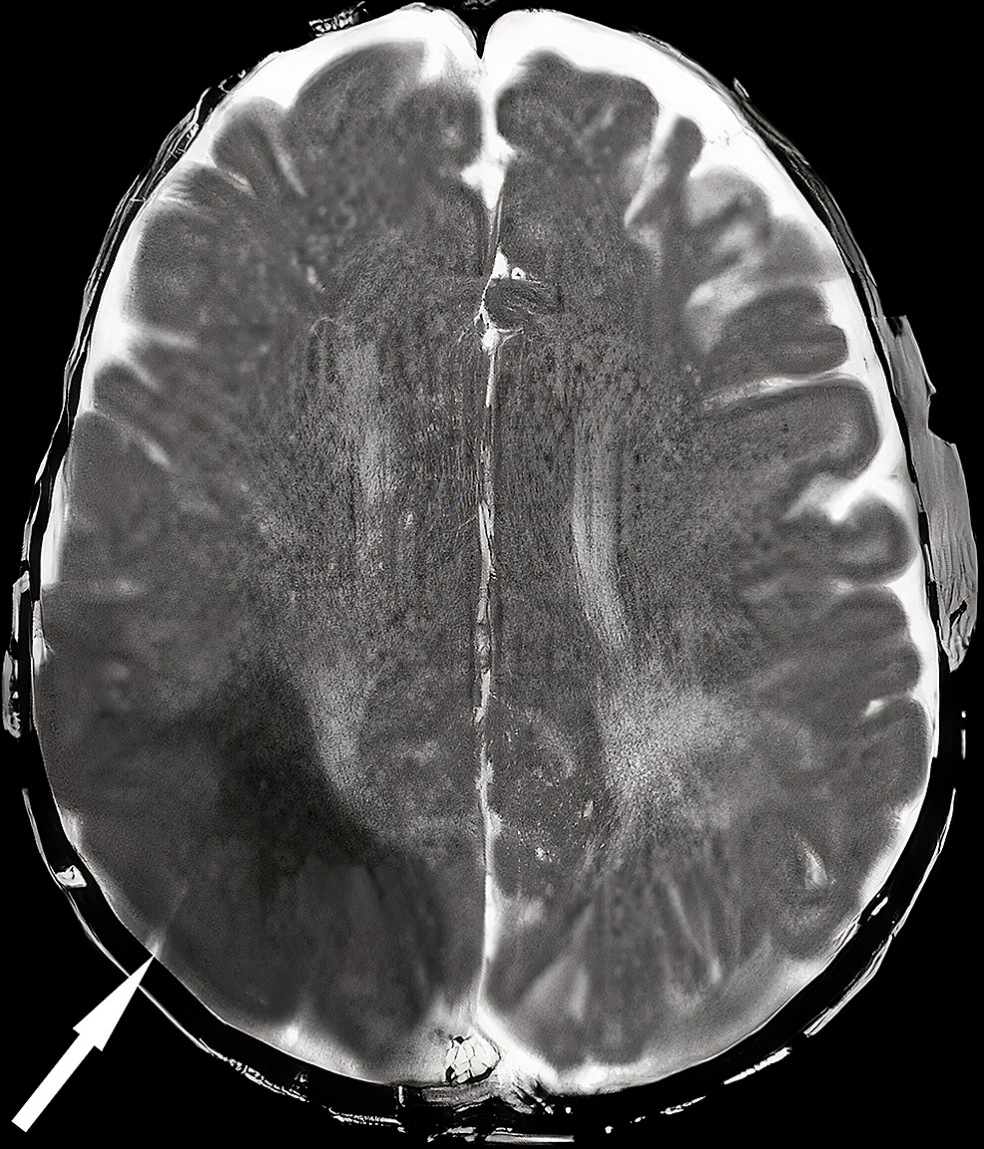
MRI shows large right parietal-temporal-occipital area cortical and subcortical area of restricted diffusion consistent with acute infarct.
